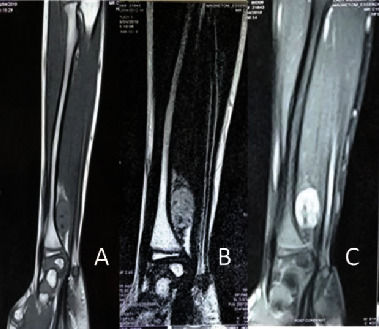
MRI showing a well-defined contrast-enhanced image of a soft tissue lesion in the forearm. (a) T1W: hypointense. (b) T2W/STIR: hyperintense. (c) Post contrast.
